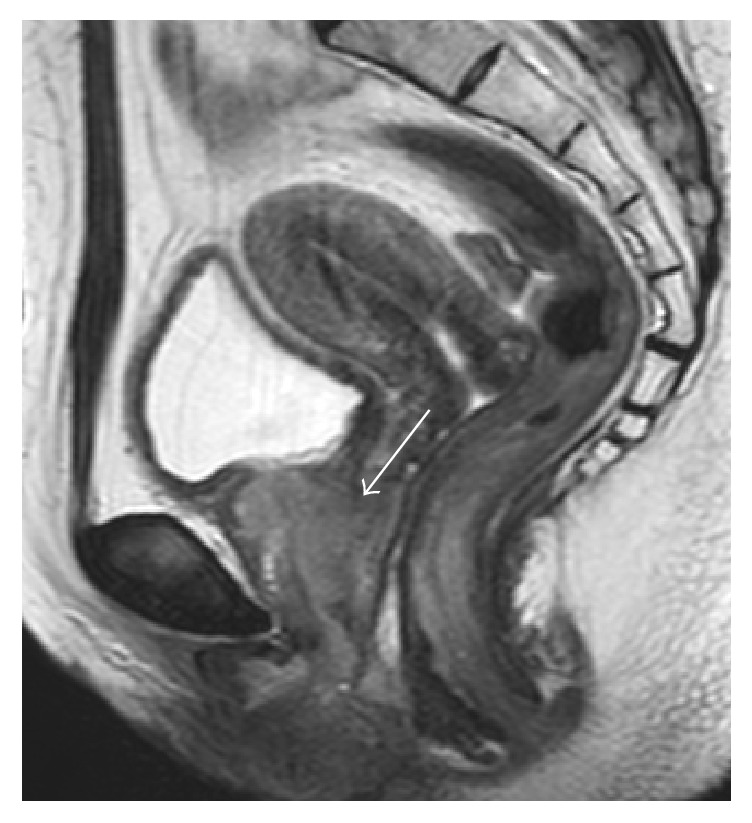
T2-weighted magnetic resonance image of the pelvis. The coronal image shows vaginal invasion of the tumor (arrow).
